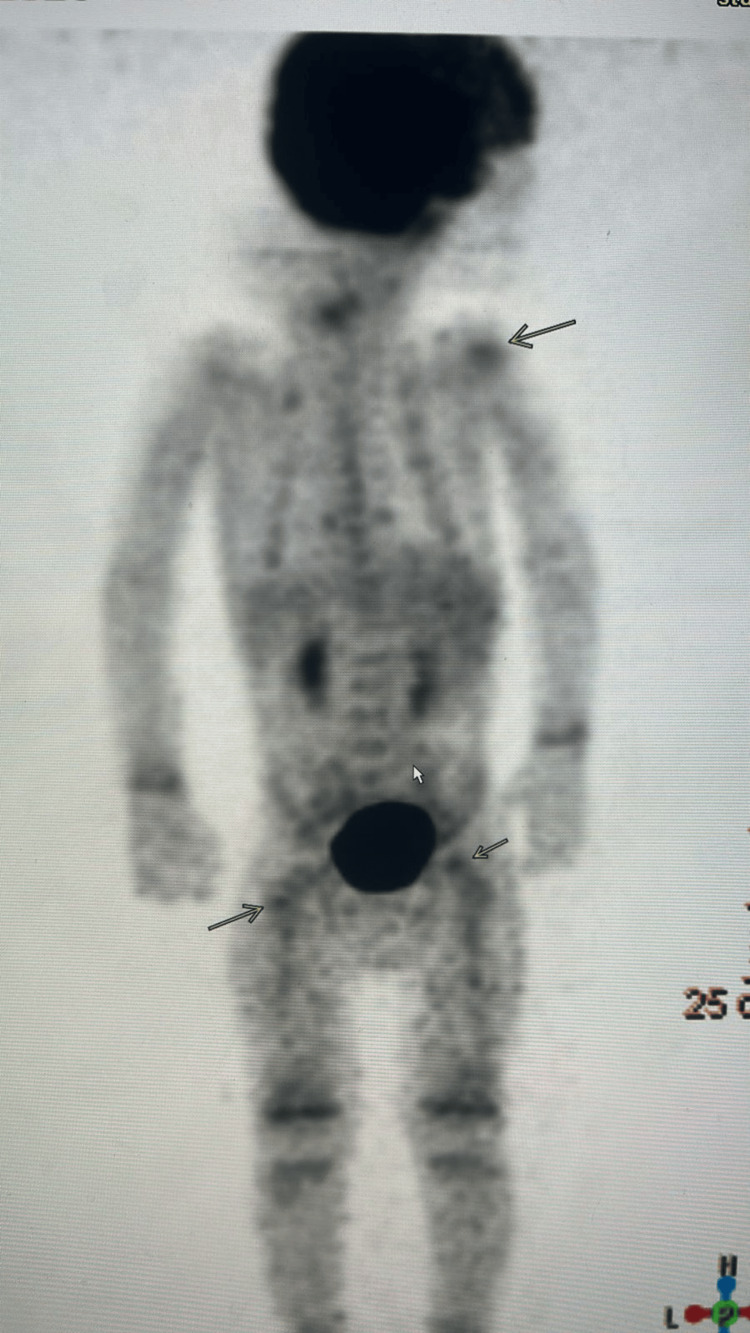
An 18-FDG PET scan showing faint uptake in the right humeral head and right femoral head (black arrows). 18-FDG PET, 18-fluorodeoxyglucose positron emission tomography
